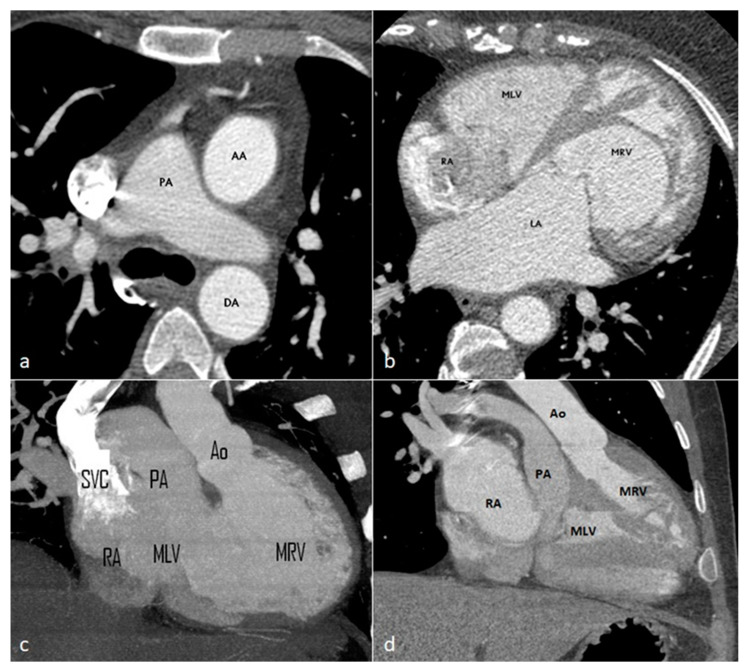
Axial (a,b) and coronal (c,d) contrast-enhanced CT images showing the levotransposition of the aorta (the ascending aorta located anterior and to the left of the main pulmonary artery) and four chambers of the heart, with the left upper cardiac border formed by the inverted aorta and anatomic right ventricular outflow tract. PA = pulmonary artery. AA = ascending aorta. DA = descending aorta. LA = left atrium. RA= right atrium. MRV = morphologic right ventricle. MLV = morphologic left ventricle. Ao = aorta. SVC = superior vena cava.
